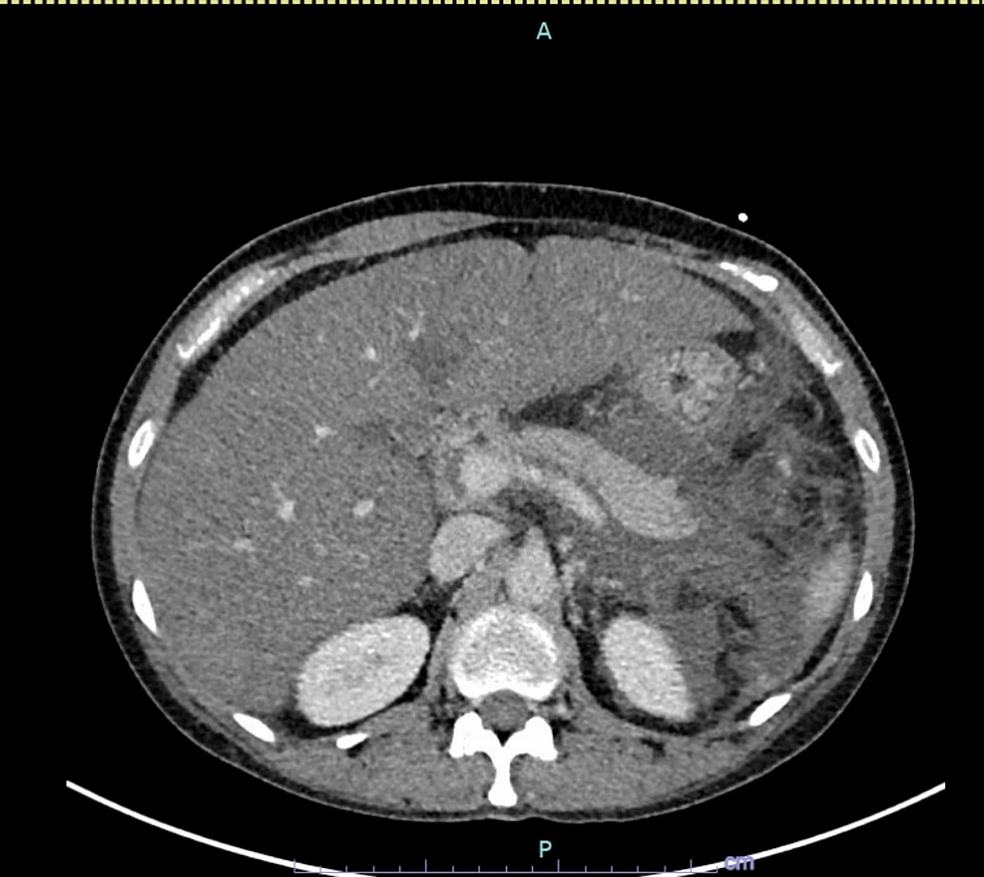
CT abdomen and pelvis with IV contrast Extensive induration of fat as well as free fluid in the left abdomen, including surrounding the pancreas, is compatible with acute pancreatitis. No loculated fluid collection or evidence of pancreatic parenchymal necrosis at this time. IV: Intravenous
